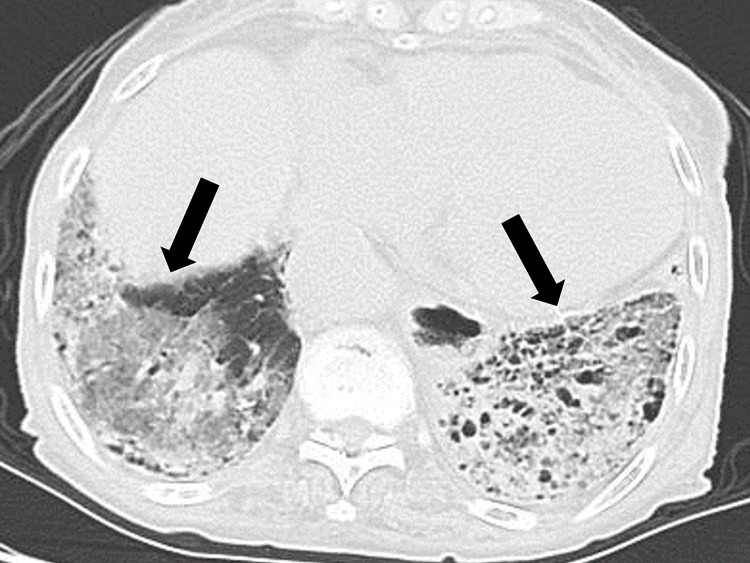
Computed tomography of the chest showing bilateral interstitial infiltration with honey-comb appearance (black arrows).
